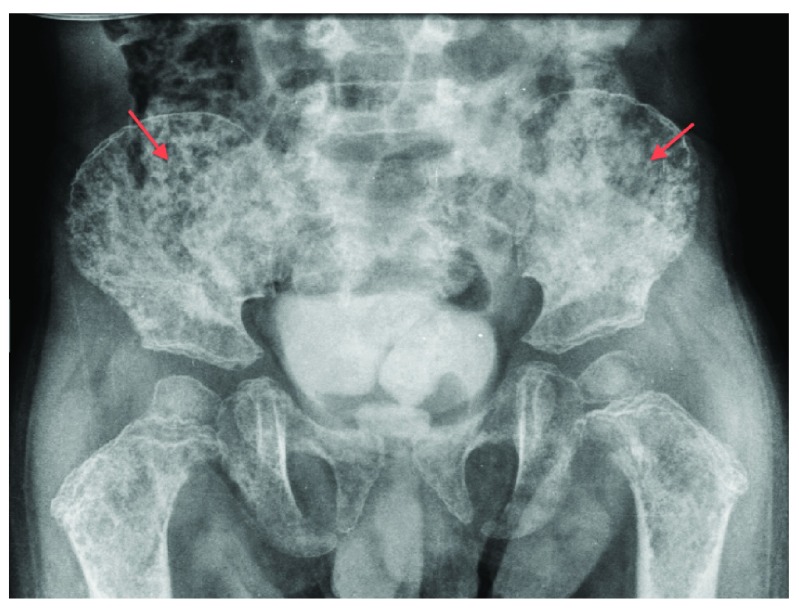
Pelvis X-ray showing osteolytic lesions secondary to bone resorption (red arrows).
